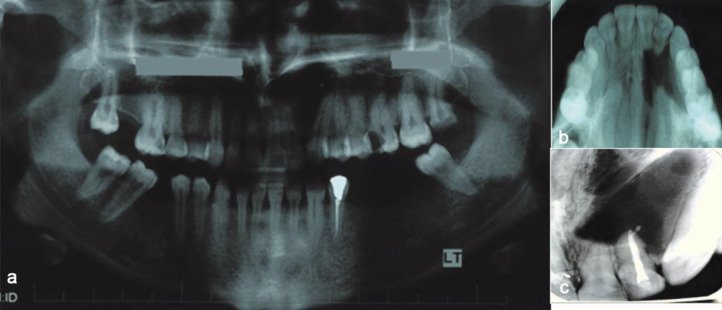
a: Periapical radiolucency around the apex of the laterla incisor (OPG), b: Periapical radiolucency around the apex of the laterla incisor (Occlusal), c: Obturation
